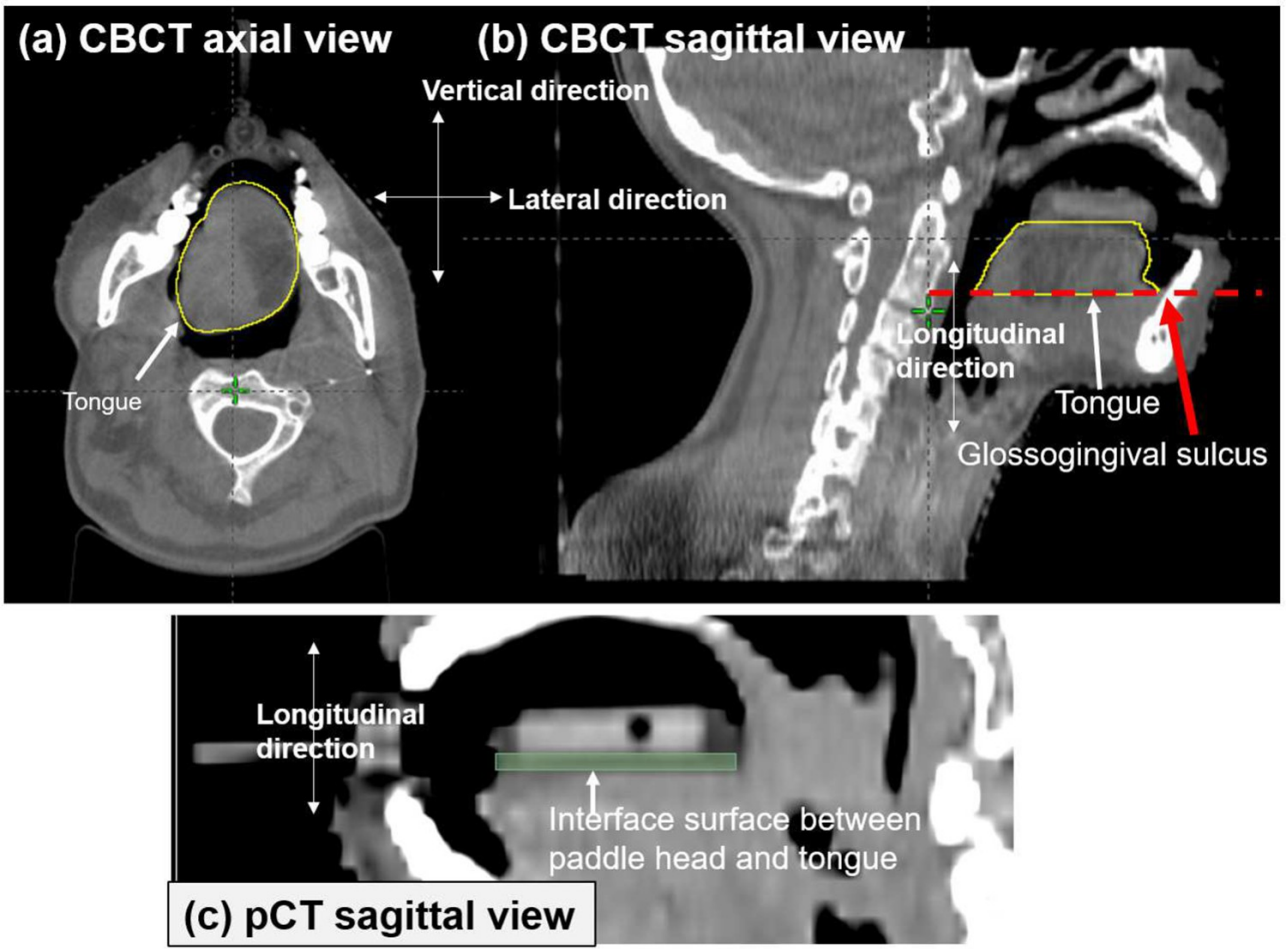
CBCT images of patients with tongue-positioning devices. (a) Axial view and (b) sagittal view of O-type tongue positioning device applied to a patient. The red dotted horizontal line shows the bottom plane of the tongue. (c) Example of contouring the surface between paddle blade and tongue for extracting the coordinates of radiation dose (RD).
